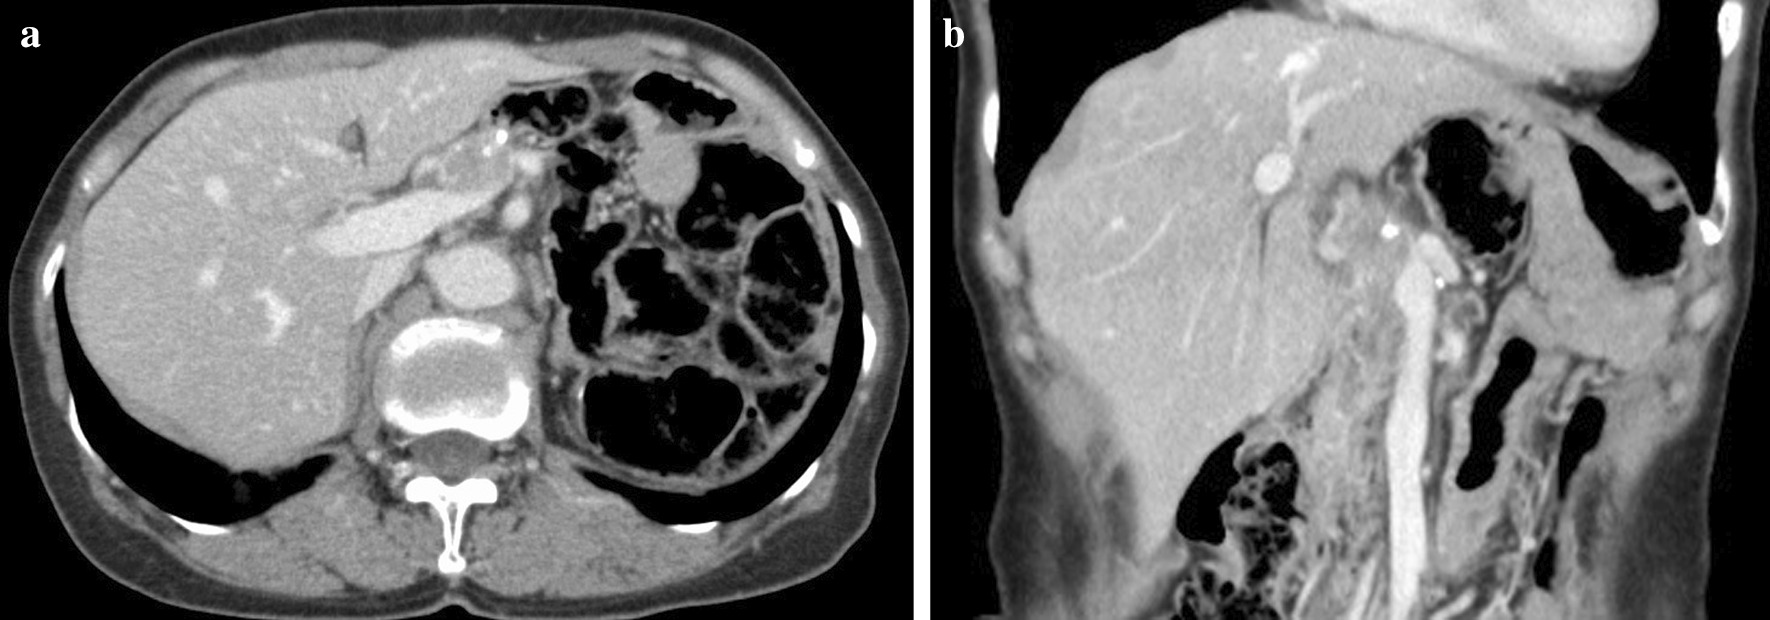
a Computed tomography (coronal plane). A nodule measuring approximately 20 mm is visible on the pancreatic stump. b Computed tomography (axial view of the same location as in Fig. 3-1)
